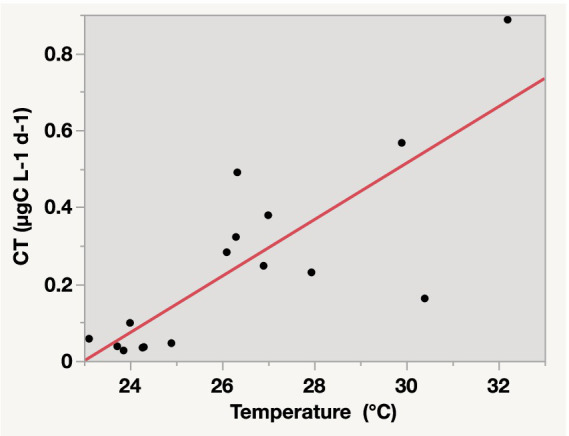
Linear relationship between carbon transfer (CT) to higher trophic levels in the microbial food web and temperature in surface waters of the Red Sea. The equation for the relationship is CT = −1.687876 + 0.0733824 × temperature, with R^2^ = 0.65 and *p-value* < 0.0001.
